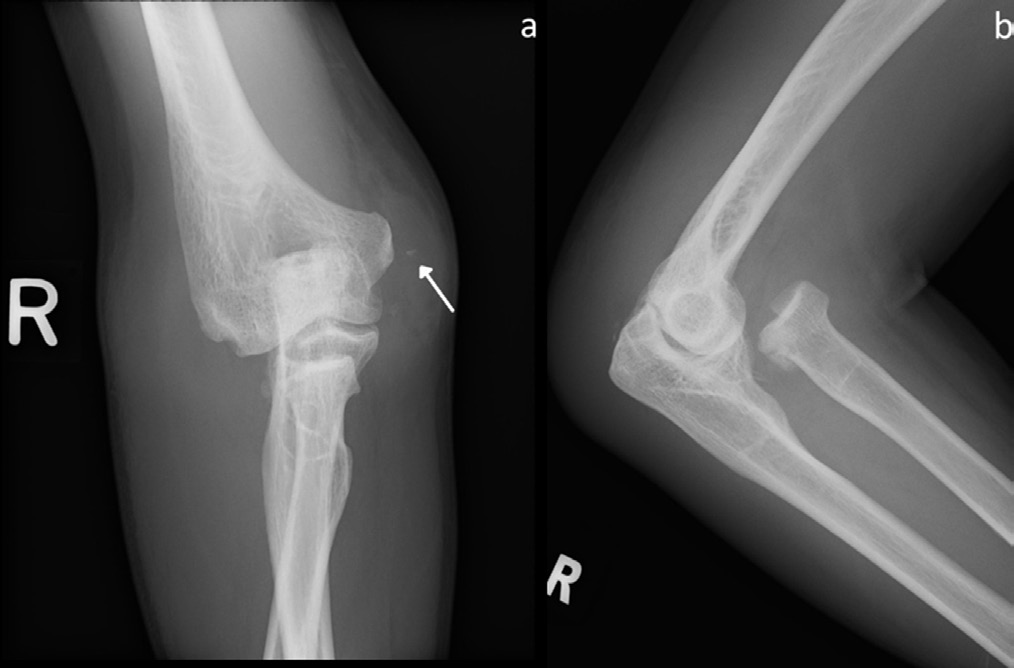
Anteroposterior (a) and lateral (b) plain radiographs of the right elbow in a 58-year-old male patient. There is a comminuted fracture of the radial head, which is also dislocated anteromedially. A tiny avulsed bone fragment is seen adjacent to the medial epicondyle (*arrow*). No ulna fracture is present.
